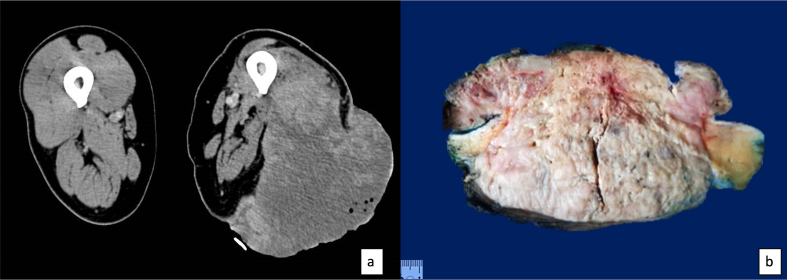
(a) CT of the right thigh, in the axial section, reveals a tumor involving the vastus lateralis and vastus intermedius muscles. The imaging shows a hyperdense peripheral component and hypodense central areas within the tumor. (b) In the cut surface, the neoplasm appears fish-like, white-pink, with hemorrhagic areas.
